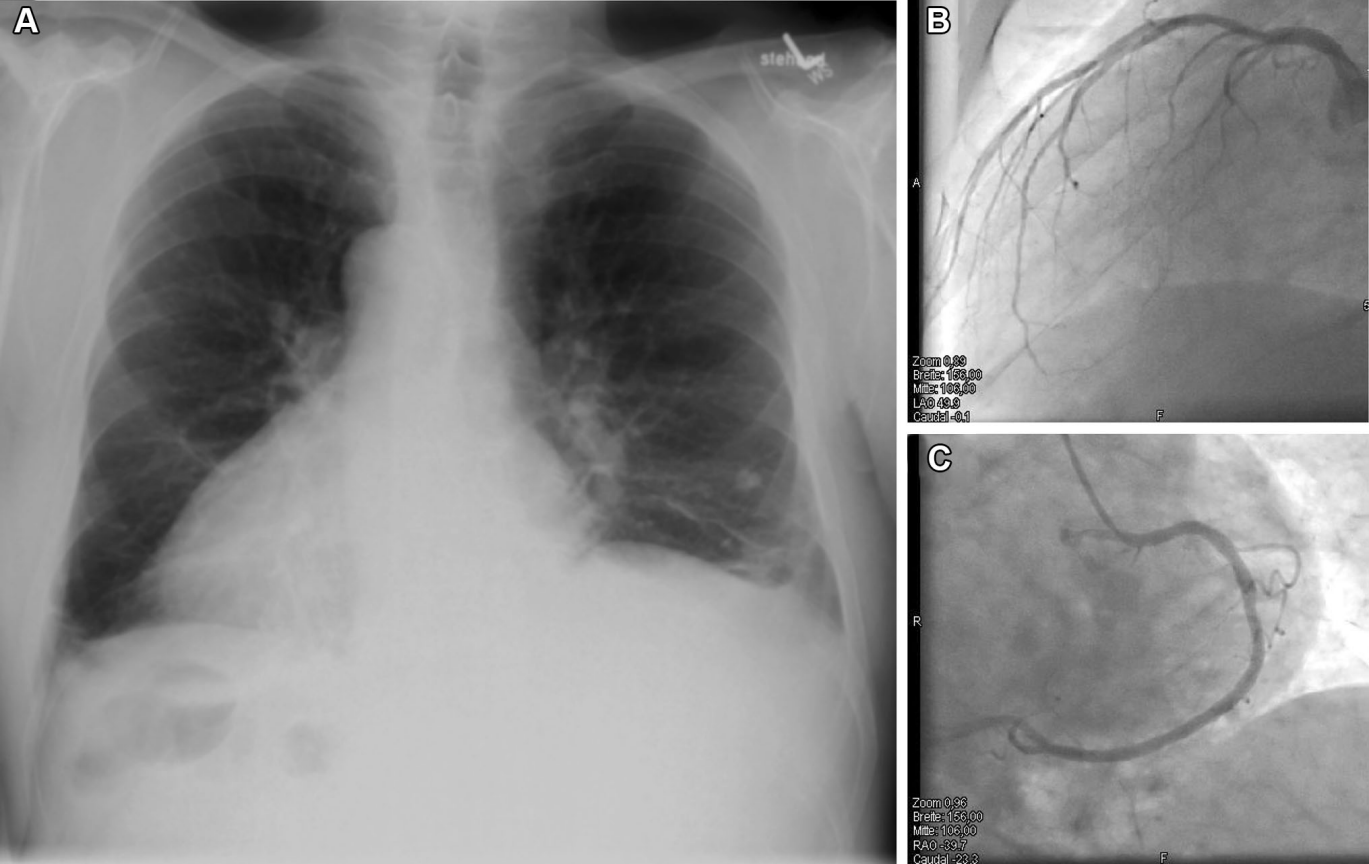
a The chest radiograph demonstrates a situs inversus totalis with dextrocardia and right-sided aortic arch. b Angiography of the left coronary artery (LAO 49.9). c Angiography of the right coronary artery (RAO −39.7)
